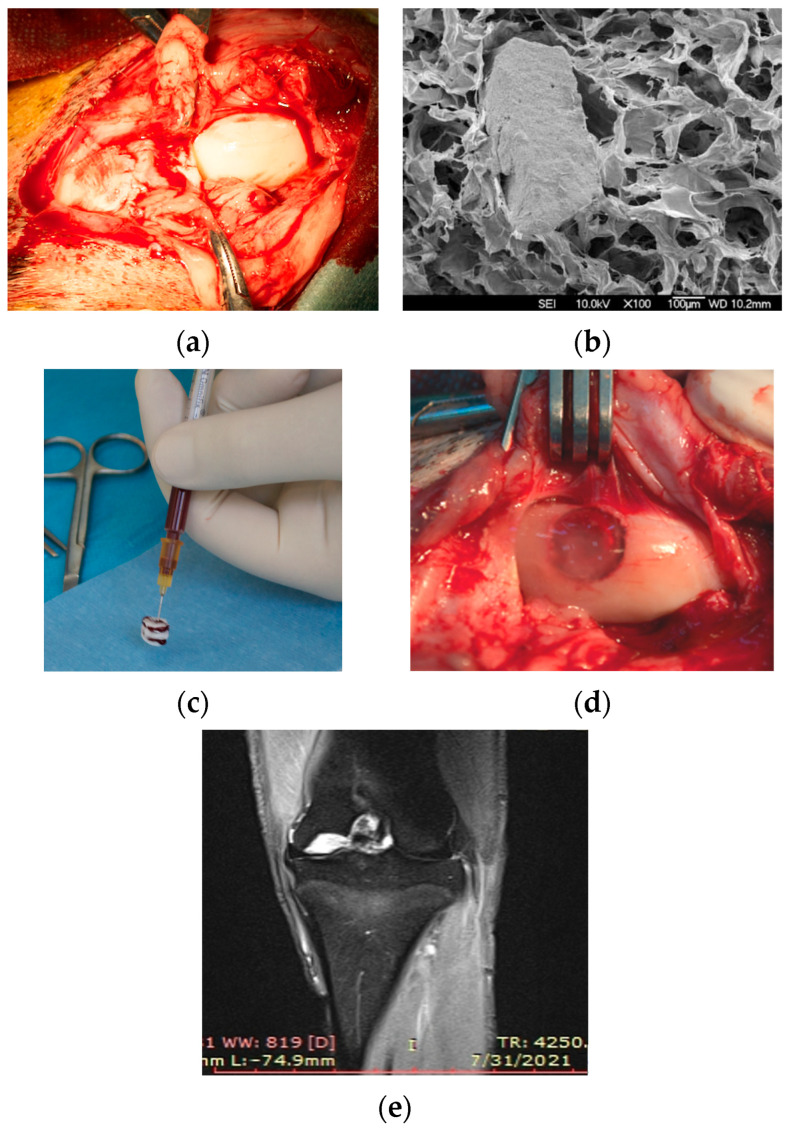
(a) Medial parapatellar approach over the left knee; (b) SEM image of porous collagen graft containing hydroxyapatite and tricalcium phosphate; (c) Injection of stem cells concentrate into the porous collagen implant; (d) Implant fixation in the defect using fibrin sealant; (e) MRI image after surgical intervention.
